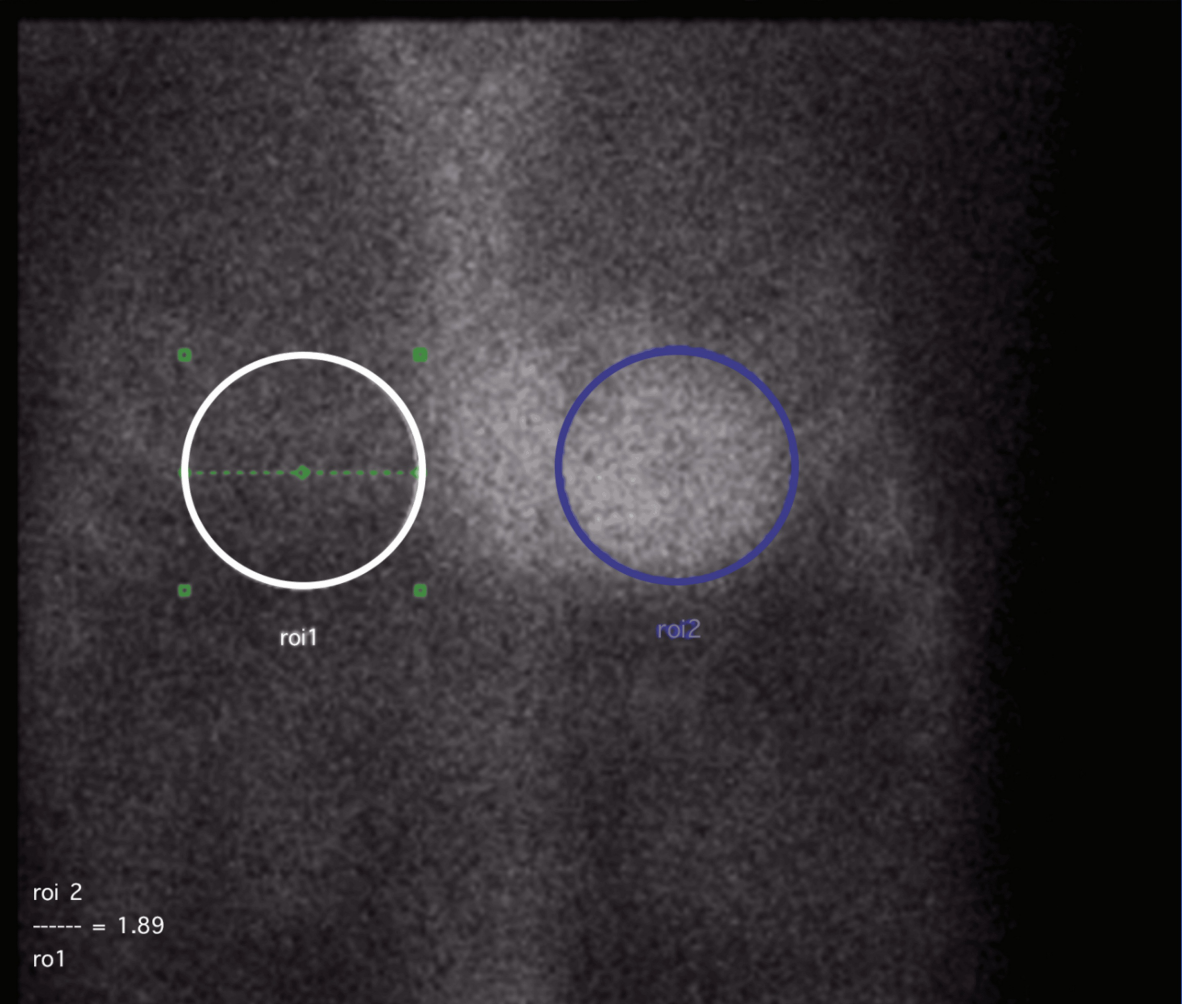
Technetium-99m pyrophosphate (PYP) scintigraphy showing strong positivity with grade 3 uptake on the Perugini scale and a heart-to-contralateral (H/CL) ratio of 1.9
